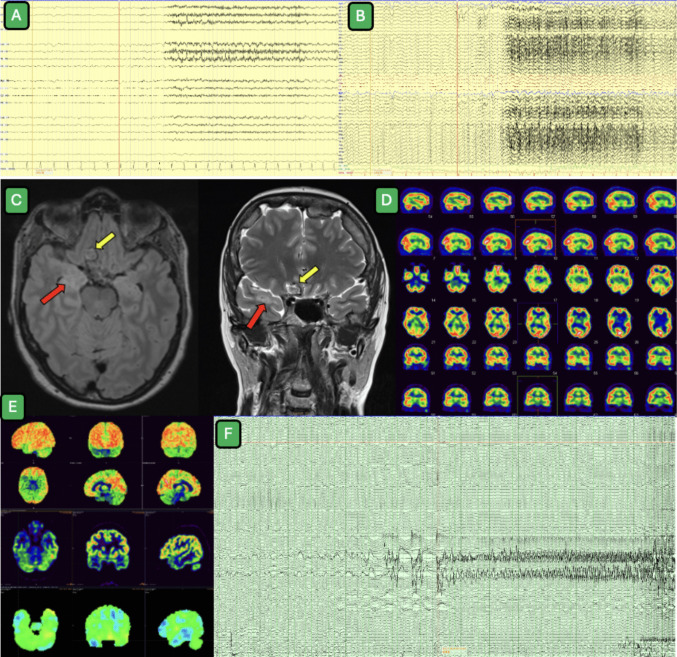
Case of a patient with drug-resistant epilepsy and dual pathology. A Bipolar EEG montage showing rhythmic spiculated activity in the right frontotemporal region. B EEG during seizure onset with progression in the right temporal chain. C MRI demonstrating right temporal sclerosis (red arrow) and right frontobasal cavernoma (yellow arrow). D SPECT with hyper uptake in the right temporal pole. E PET showing hypometabolism in right temporal and anterobasal frontal regions. F SEEG confirming seizure onset in hippocampal contacts O1-2 and H1-2, leading to ipsilateral temporal lobectomy via an endoscopic transorbital approach
